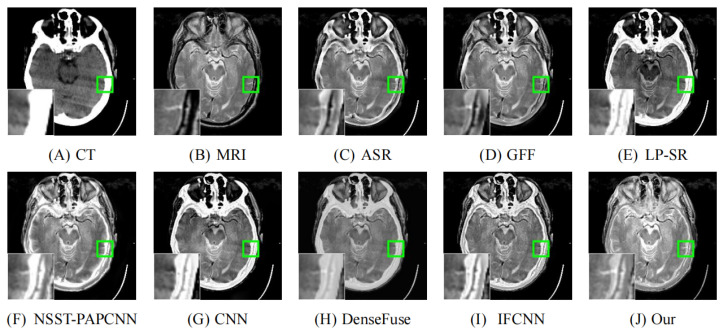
Fusion images of “Meningioma-7” by various methods. From (A) to (J): CT, MRI, ASR, GFF, LP-SR, NSST-PAPCNN, CNN, DenseFuse, IFCNN, Our proposed method.
